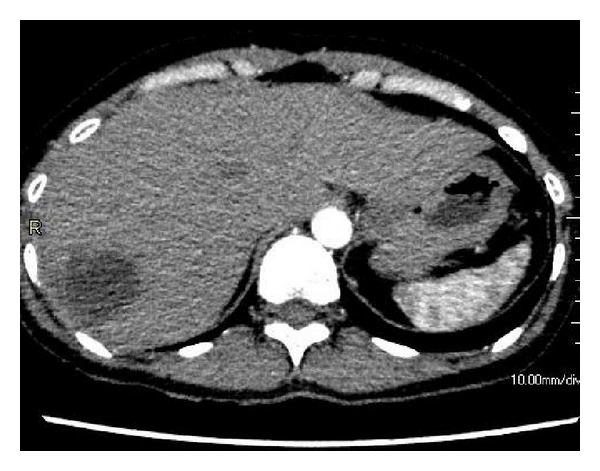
Follow-up CT performed on day 58. The size of the low-density area of the anterior segment of the liver is reduced, and the arterioportal fistula has disappeared.
